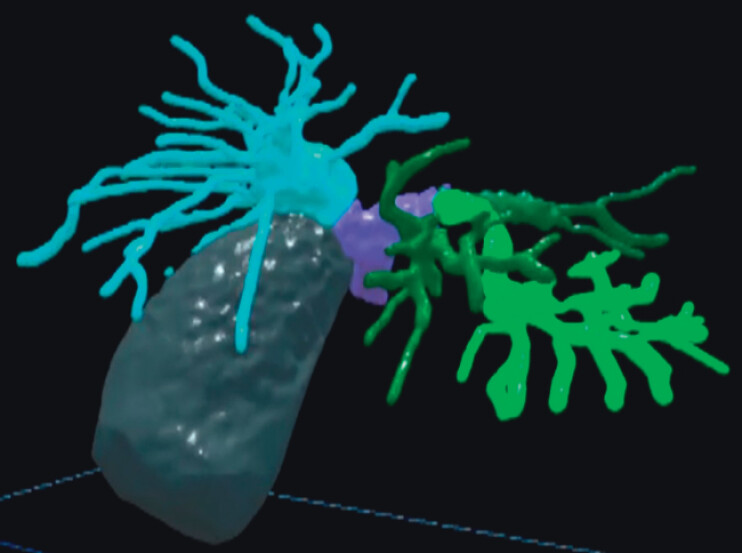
Computed tomography-derived virtual reality biliary model. Organ filters enable selective visualization of specific organs or regions, and color-coding facilitates intuitive understanding of anatomical relationships.
